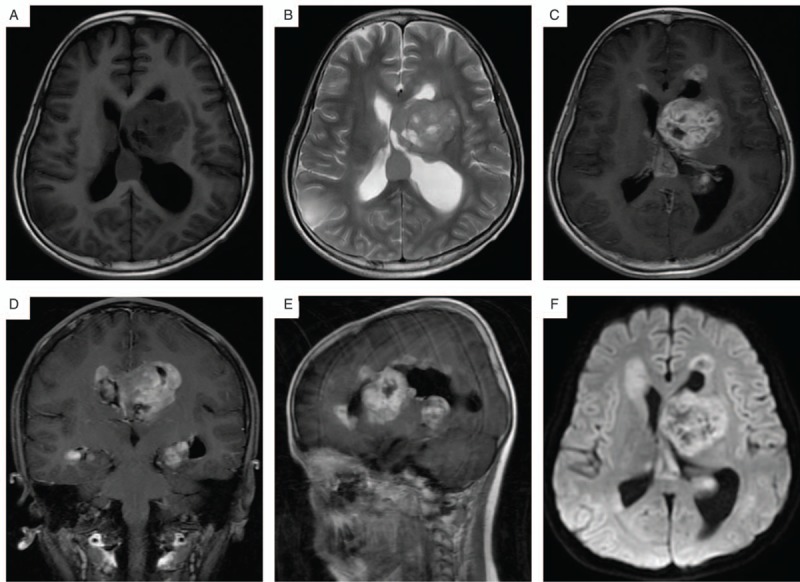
Follow-up magnetic resonance imaging (MRI) after 10 months from operation demonstrates intracranial metastasis. (A, B) T1-weighted and T2-weighted axial MR images show new lesions located in lateral cerebral ventricle and enlargement of ventricle. (C) T1-weighted sagittal MR image with contrast reveals heterogeneous enhancement. (D, E) Coronal and sagittal sections display several metastases. (F) Apparent diffusion coefficient (ADC) mapping shows heterogeneous signal.
